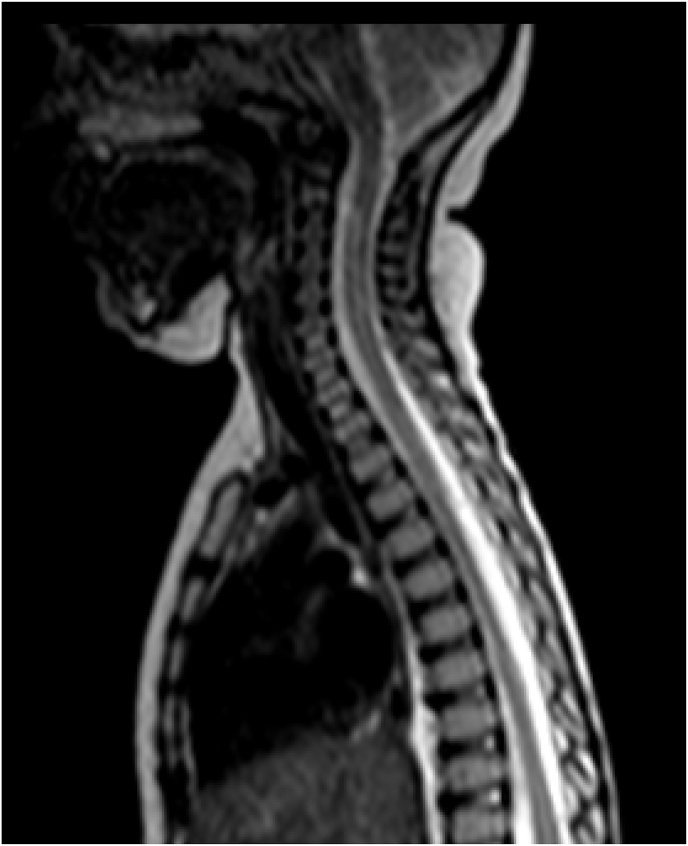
MRI T2 Sagittal showed partial resolution of the changes seen previously.
